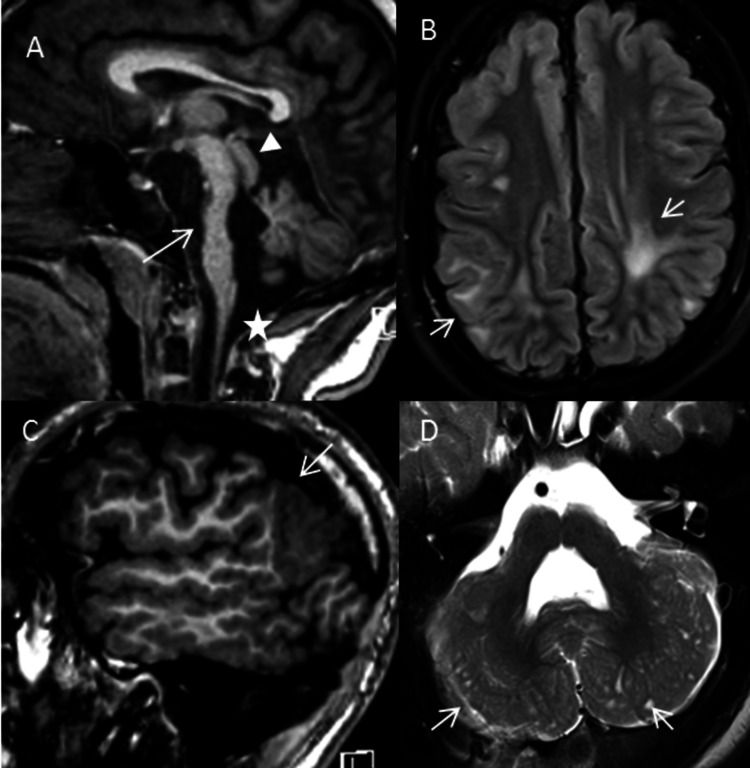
Fukuyama congenital muscular dystrophy (FCMD) A: Sagittal T1 MRI revealing marked hypoplasia of the pons (arrow) with inferior vermian hypoplasia (star) and fused midbrain colliculi (arrowhead). B: Axial FLAIR image showing patchy areas of white matter hyperintensity (arrows). C: Extensive polymicrogyria is noted in the frontal, parietal, and temporal lobes (arrow) on the T1 sagittal image. D: Axial T2 image showing multiple tiny cysts (arrows) adjacent to the dysplastic cerebellar cortex.
